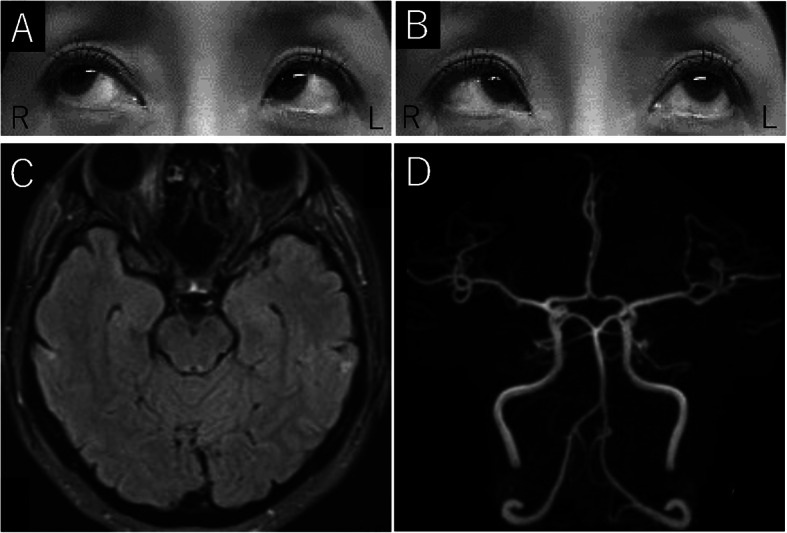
a, b Case 2: Limited abduction of the left eye. c, d Case 2: FLAIR images and MRA show no abnormalities
